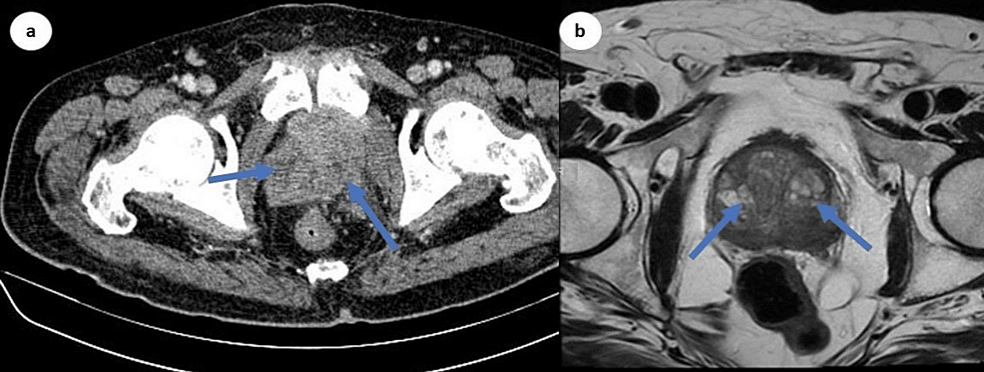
CT (a) and MRI (b) demonstrating multiple ill-defined lesions in the anterolateral regions of the prostate with irregular borders. CT: computed tomography; MRI: magnetic resonance imaging
